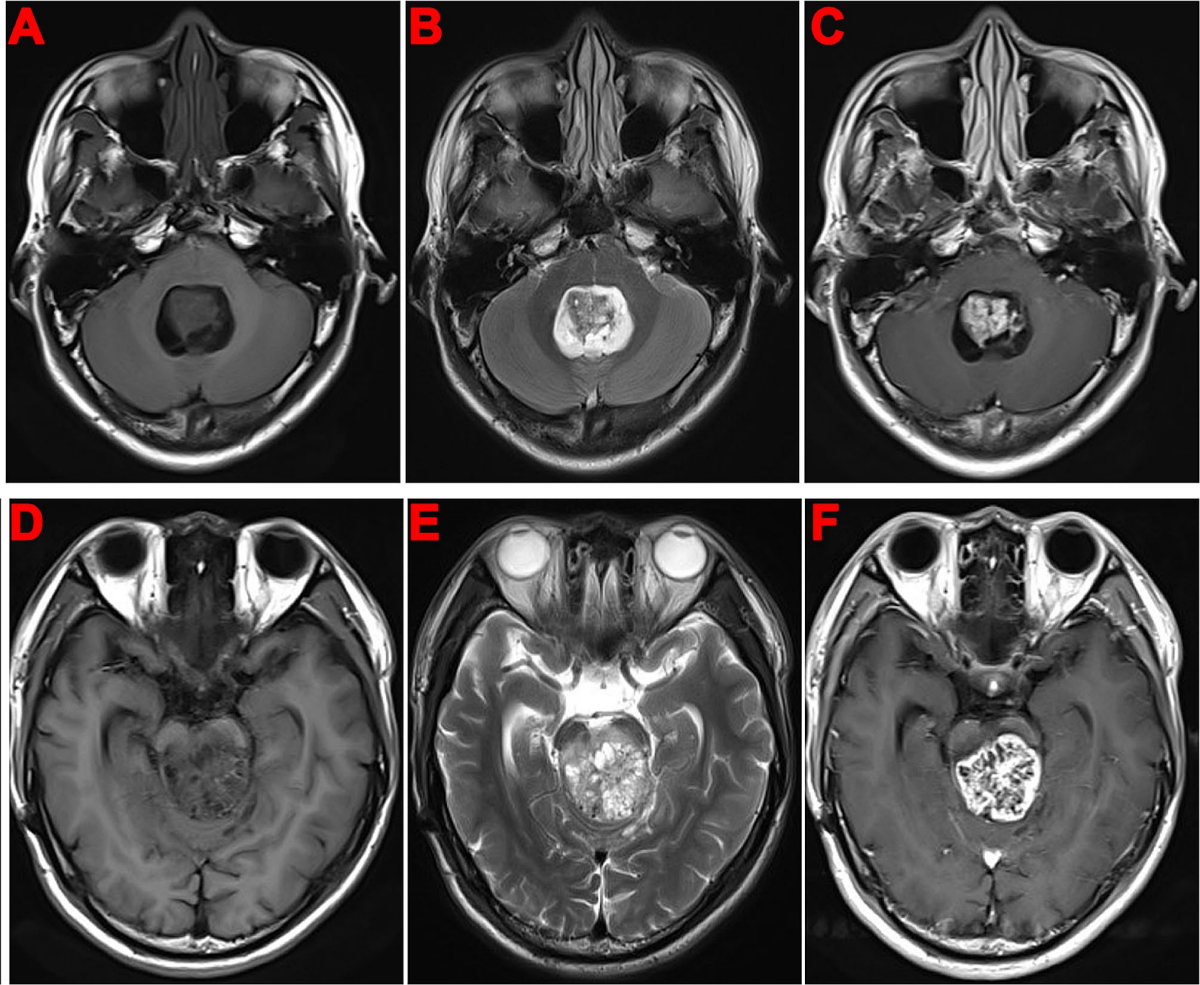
Head MRI: A-C, In case 2, the fourth ventricle was enlarged, containing an irregular tumor with an abnormal signal. The tumor displayed mixed medium and low signals on T1WI, with mixed high signals on T2WI. The lesion center appeared as an isointense signal. Contrast-enhanced scanning revealed significant enhancement within the substantial region of the lesion, and slight cystic changes were observed; D-F, In case 4, a round space-occupying lesion was identified in the brainstem. T1WI revealed an uneven low signal, and T2WI displayed an uneven high signal. Multiple small cystic changes were observed at the edge of the lesion. On plain scanning, the lesion border was unclear, and mild edema was present. Following enhanced scanning, the lesion demonstrated an uneven enhancement, with the cystic part unenhanced and the lesion border clearer
